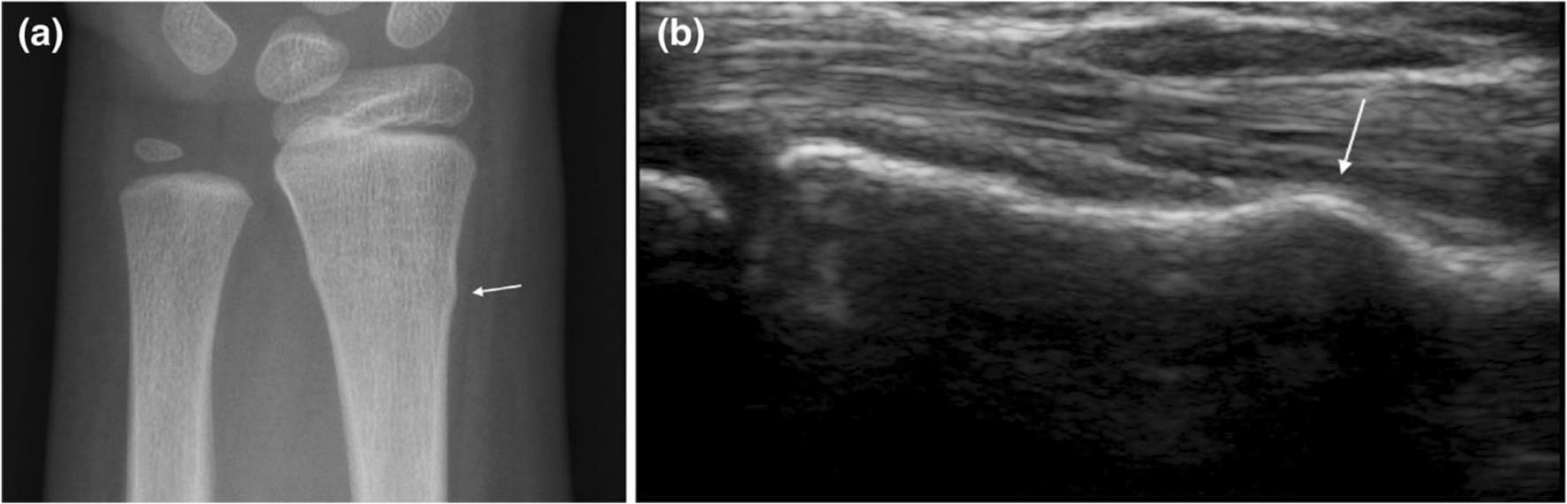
a Radiograph of a distal radius type A buckle fracture (arrow). b Ultrasound image of a distal radius buckle fracture (arrow) on the same patient (Snelling, 2018). Reprinted with permission from Wiley Online Library [19]
